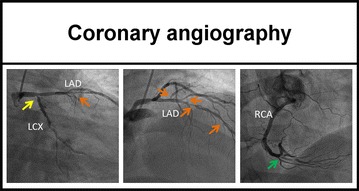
Coronary angiography. Coronary angiography revealed multi-vessel disease with a severe (76%-95%) ostial lesion of the circumflex artery (*yellow arrow*), 2 successive severe (76%-95%) medial lesions of the left anterior descending artery, a severe (76%-95%) ostial first and second diagonal’s lesions (*orange arrows*), and finally a severe (76%-95%) ostial lesion of a small posterior descending artery (*green arrow*). The extent of the coronary disease was better identified by the CardioPET than by the Tc-99m-Sestamibi perfusion SPECT
